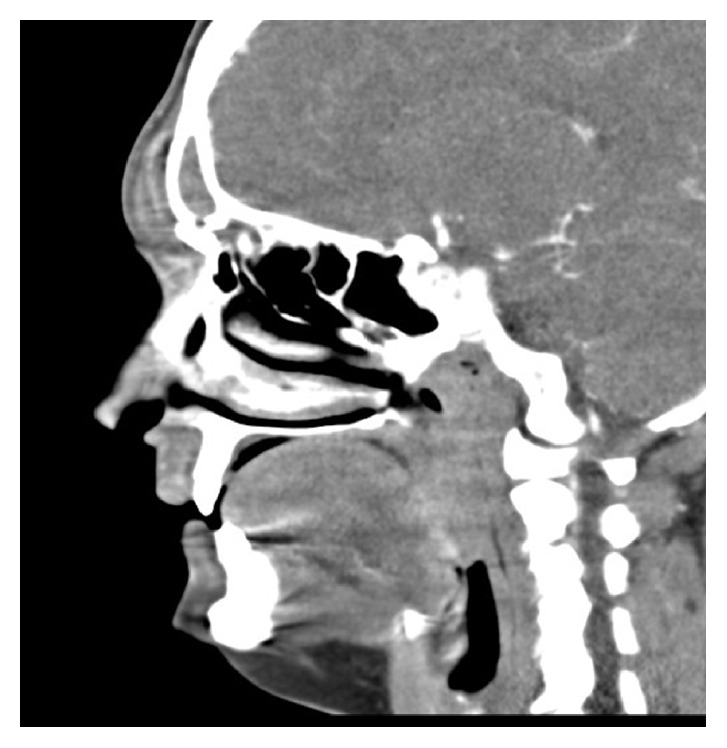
Sagittal CT image (mucocele overlying frontal sinus).
